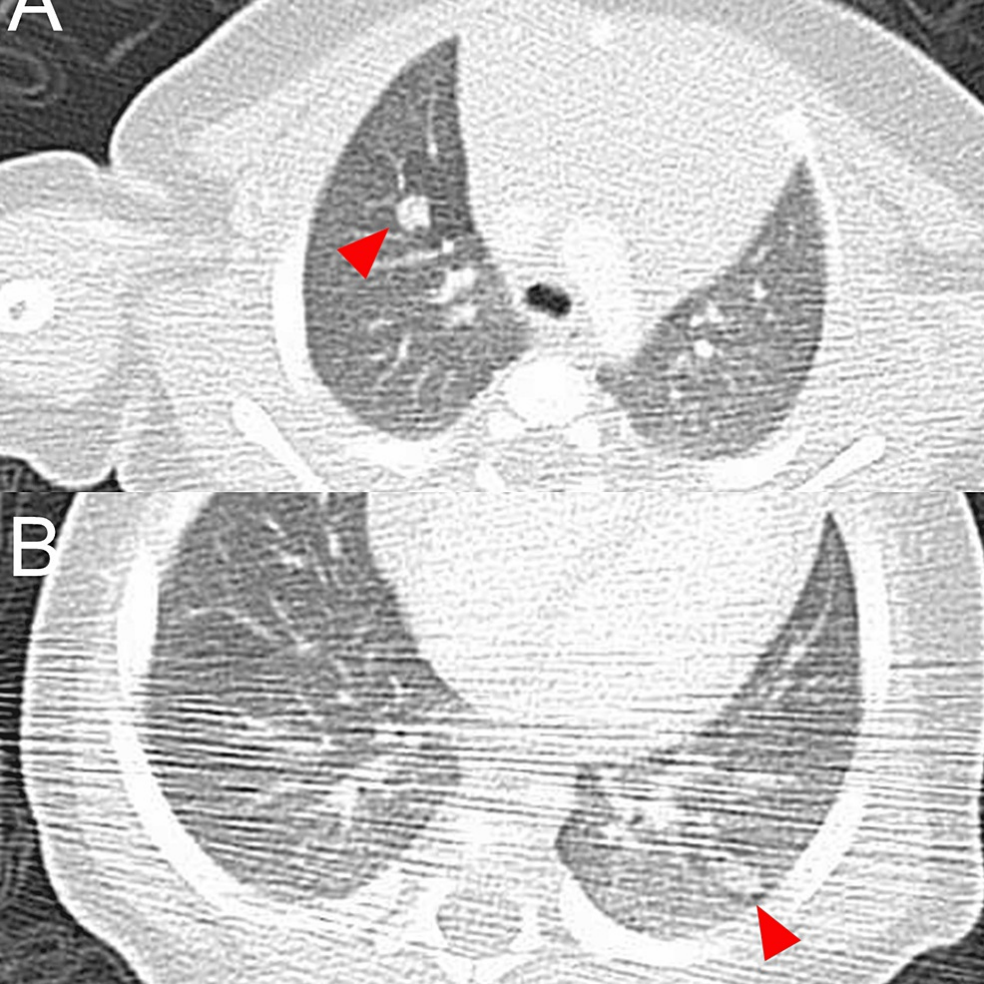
Computed tomography examination A chest computed tomography imaging revealed clearly demarcated multiple nodules in both lung (A and B). Red arrow heads indicate the nodules in the lung.
